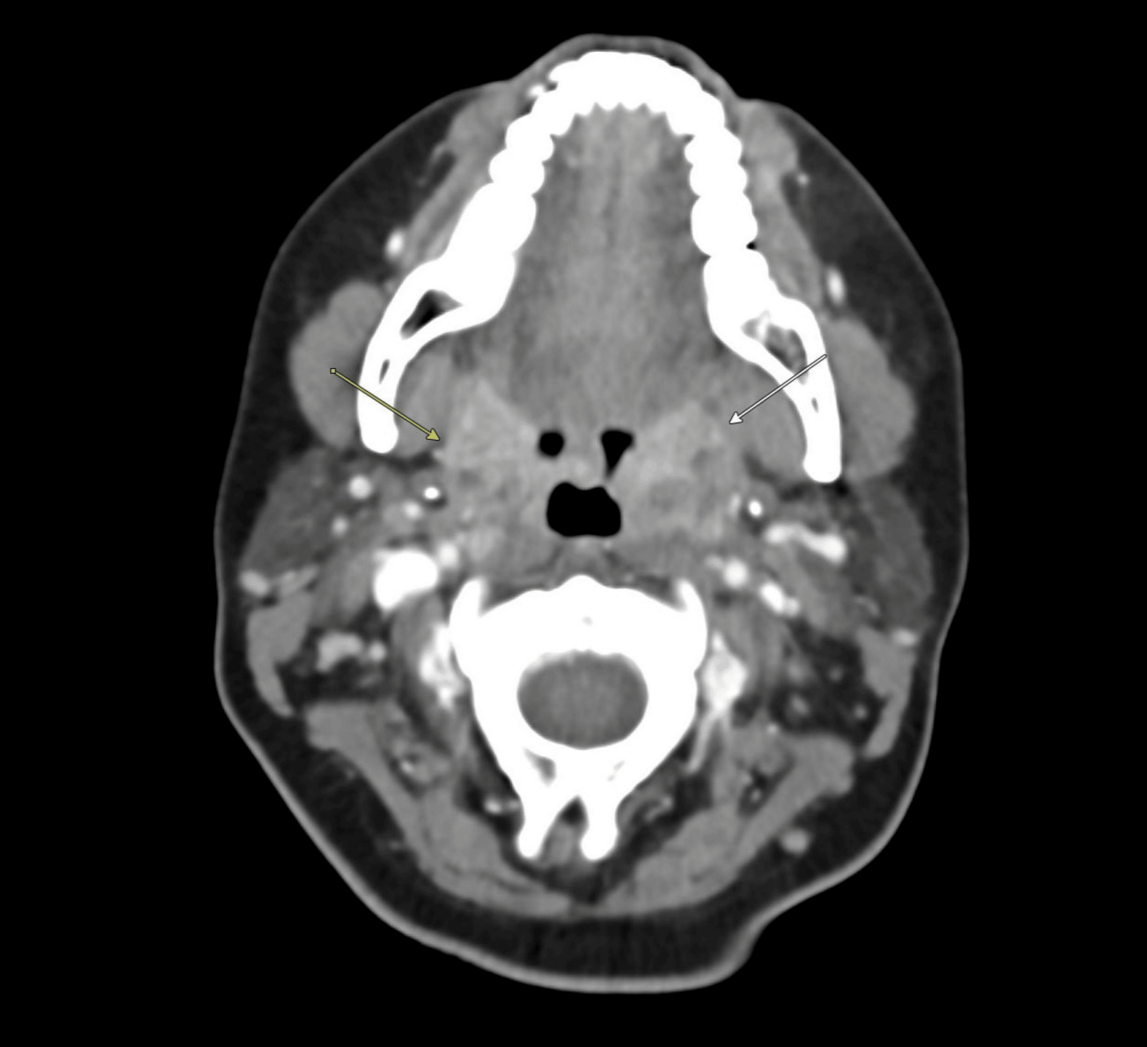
Transverse computed tomography image of the neck demonstrating bilateral palatine tonsillar enlargement consistent with acute tonsillitis (arrows)
